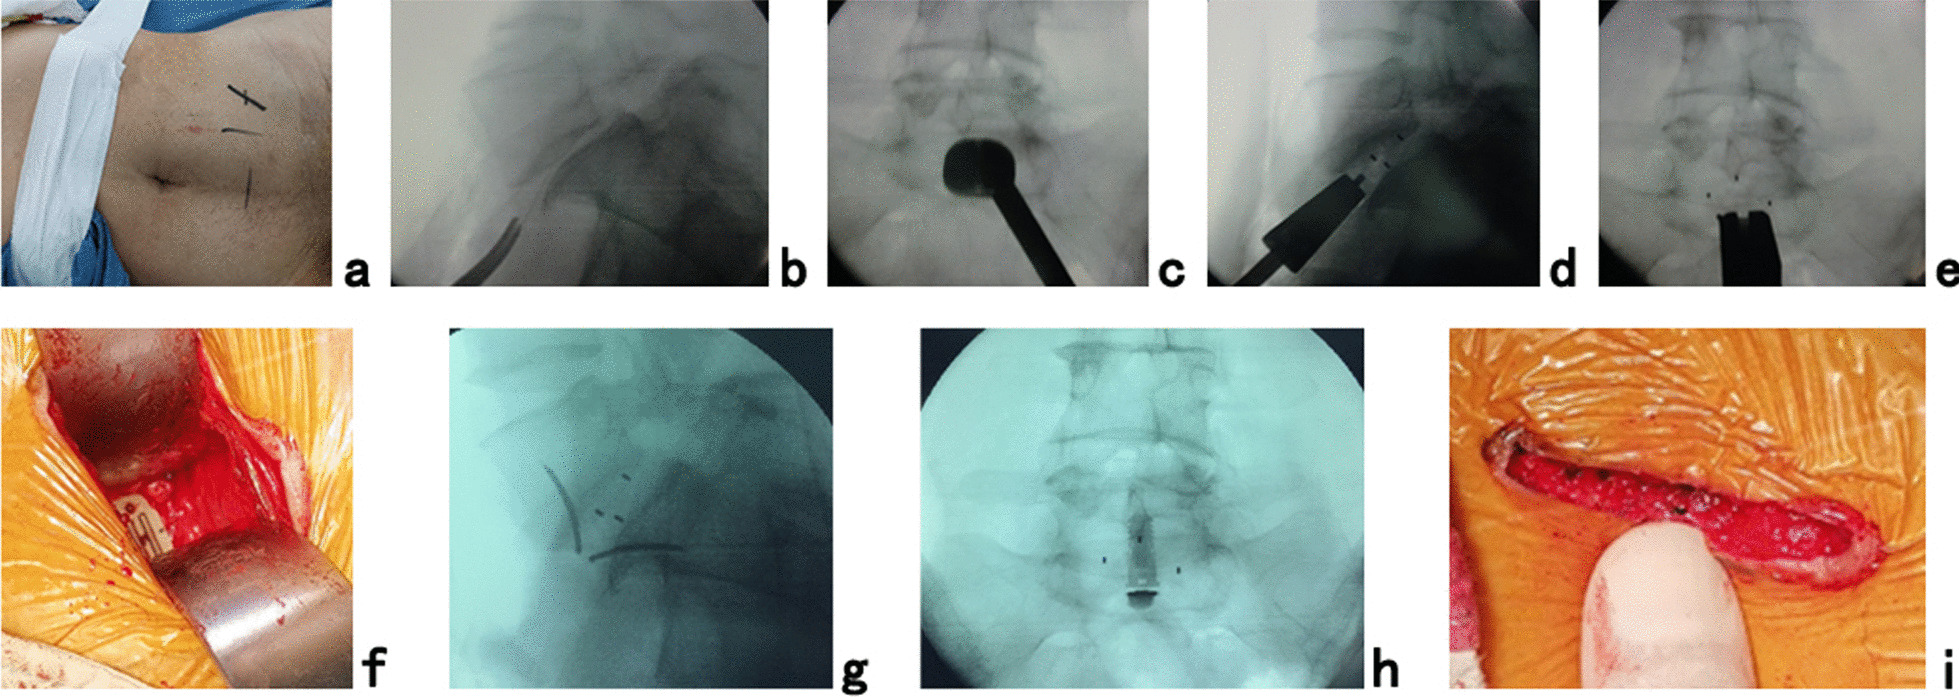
OLIF with a self-lock cage through a mini-incision for the surgical treatment of L5 spondylolisthesis. a The patient was placed into a right oblique position. The L5/S1 intervertebral space was positioned using b C-arm view. c X-ray view showing trial molding after discectomy. d Lateral and e posteroanterior C-arm views were used to check the position of the self-lock cage placed into the disk space parallel to the endplate. f The picture shows the surgical field after two self-lock anchors were inserted from the cage into the vertebrae. g Lateral and h posteroanterior C-arm views confirmed good positioning of the internal instruments. i The photograph shows the mini-incision for OLIF (Case 3)
